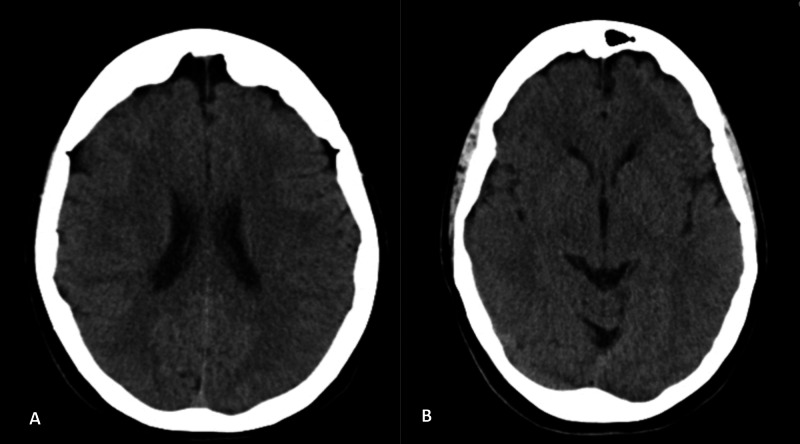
Brain CT of the patient Panel A and panel B show an expected brain CT for the age and gender of the patient, without any clinically relevant alteration CT: computed tomography
